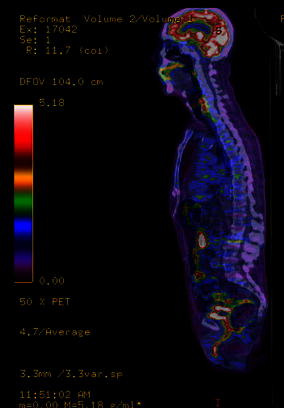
PET-CT section 9 months after endovascular abdominal aortic aneurysm repair was not significative of infection
